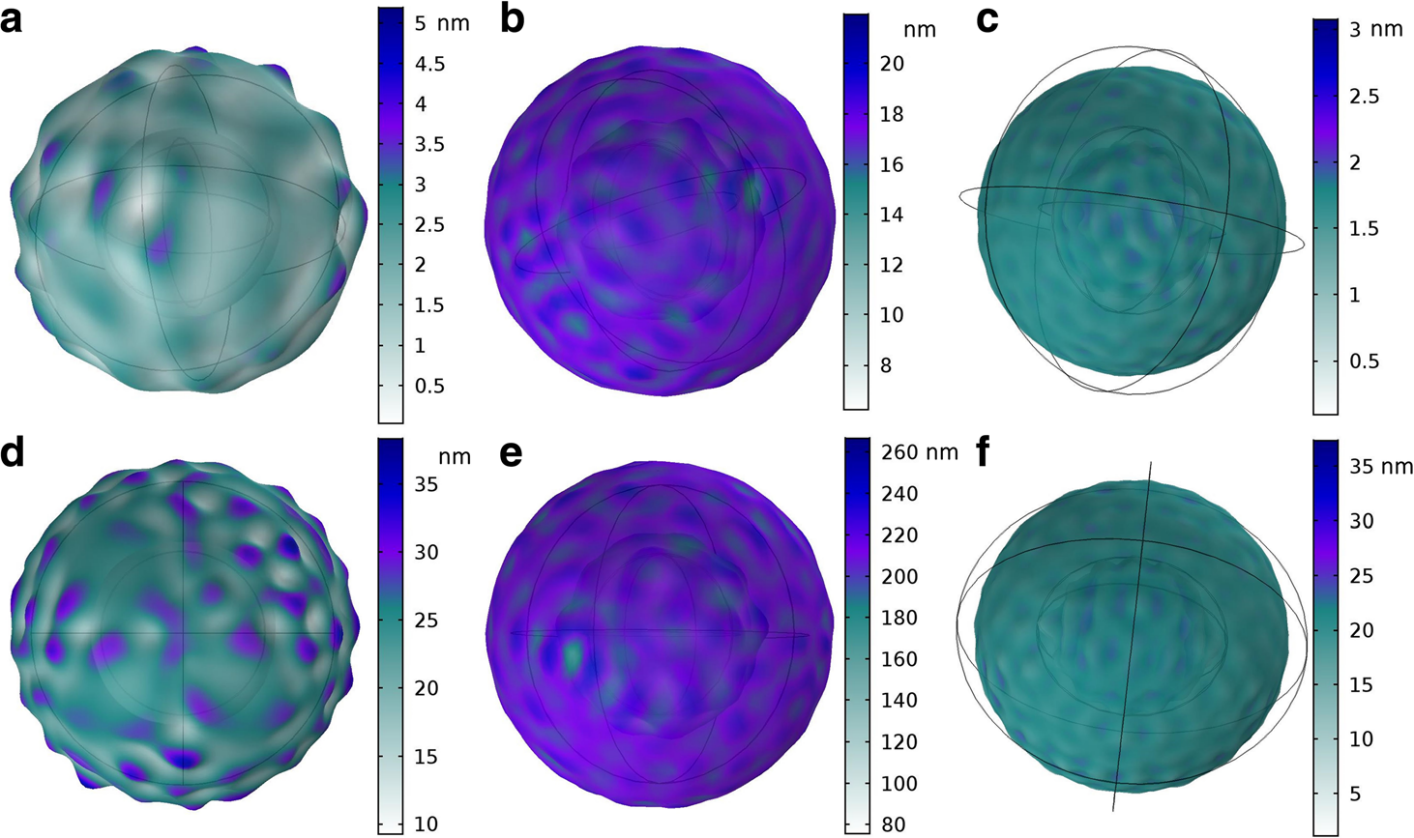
Ultrasound induced cellular deformation, the color represents displacement in nanometers. The frequency and pressure amplitude was varied a) 1 MHz; 14 kPa, b) 5 MHz; 14 kPa, c) 6.5 MHz; 14 kPa, d) 1 MHz; 170 kPa, e) 5 MHz; 170 kPa, f) 6.5 MHz; 170 kPa. (The knobby appearances in A and D are exaggerated to visually see the displacement. The displacement magnitude is depicted by color and is not depicted to scale in the figures)
